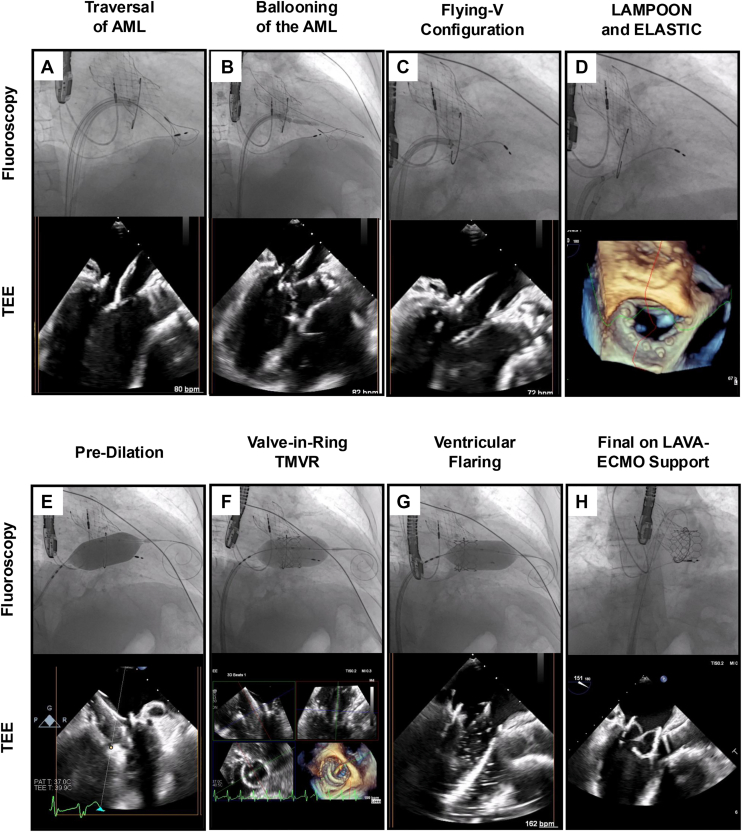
Procedural steps on fluoroscopic and TEE imaging. (A) Traversal of AML with electrified Astato wire. (B) Ballooning of the AML. (C) Flying-V configuration. (D) LAMPOON and ELASTIC. (E) Pre-dilation of the mitral valve annulus. (F) Valve-in-Ring TMVR. (G) Ventricular flaring. (H) Final on LAVA-ECMO support. AML, anterior mitral leaflet; ECMO, extracorporeal membrane oxygenation; ELASTIC, electrosurgical laceration of Alfieri stitch; LAMPOON, laceration of the anterior mitral valve leaflet to prevent outflow obstruction; LAVA, left atrial venoarterial; TEE, transesophageal echocardiogram; TMVR, transcatheter mitral valve replacement.
